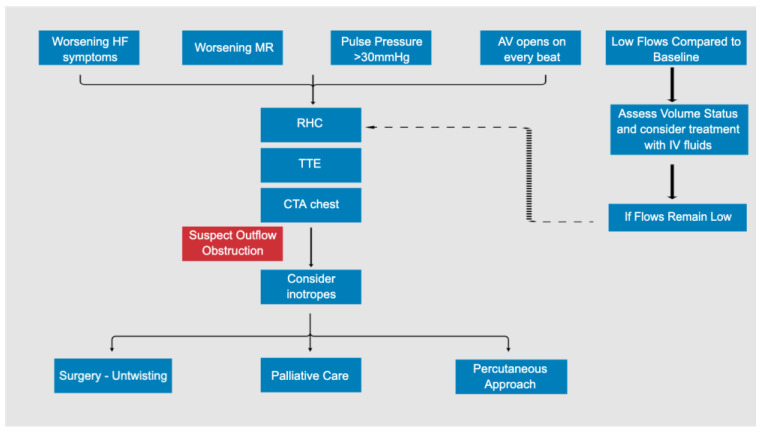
Algorithm for the evaluation of LVAD outflow graft obstruction. AV, aortic valve; CTA, computed tomography with angiography; HF, heart failure; IV, intravenous; MR, mitral regurgitation; RHC, right heart catheterization; TTE, transthoracic echocardiogram.
